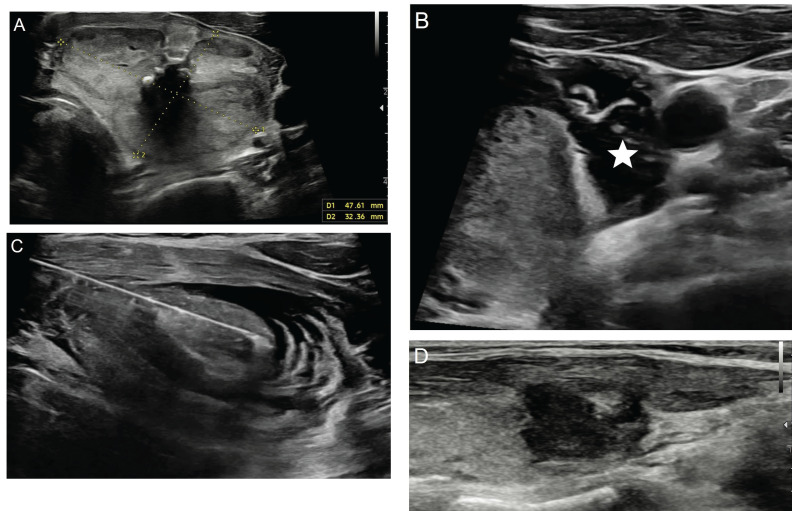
Ultrasonography image of a 57-year-old male patient show a benign solid nodule (A) measuring 47x32 mm in the left lobe of the thyroid gland, as confirmed by two separate pathological examinations. The patient underwent hydrodissection (B, asterix) followed by percutaneous microwave ablation with the trans-isthmic approach (C) using 17G uncooled system (TATO, Terumo, Japan). In the first year control ultrasonography image, a significant loss in size and echogenicity is observed in the nodule (D).
